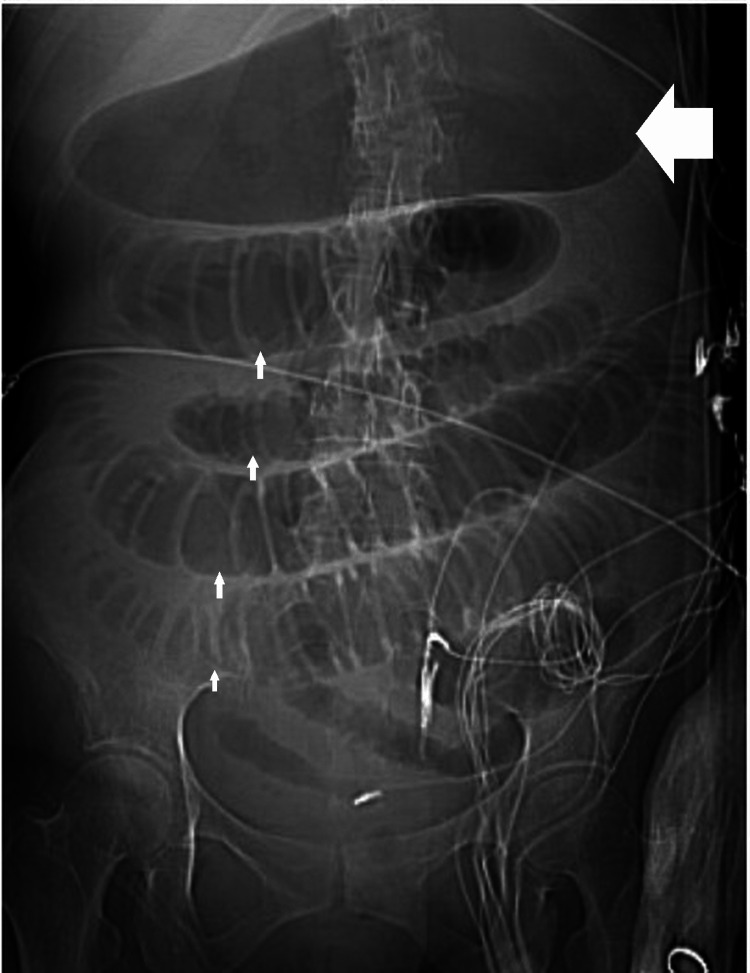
Scout film from the abdomen/pelvis CT revealed distention of the stomach (horizontal arrow) and distended small bowel loops (vertical arrows) consistent with small bowel obstruction
